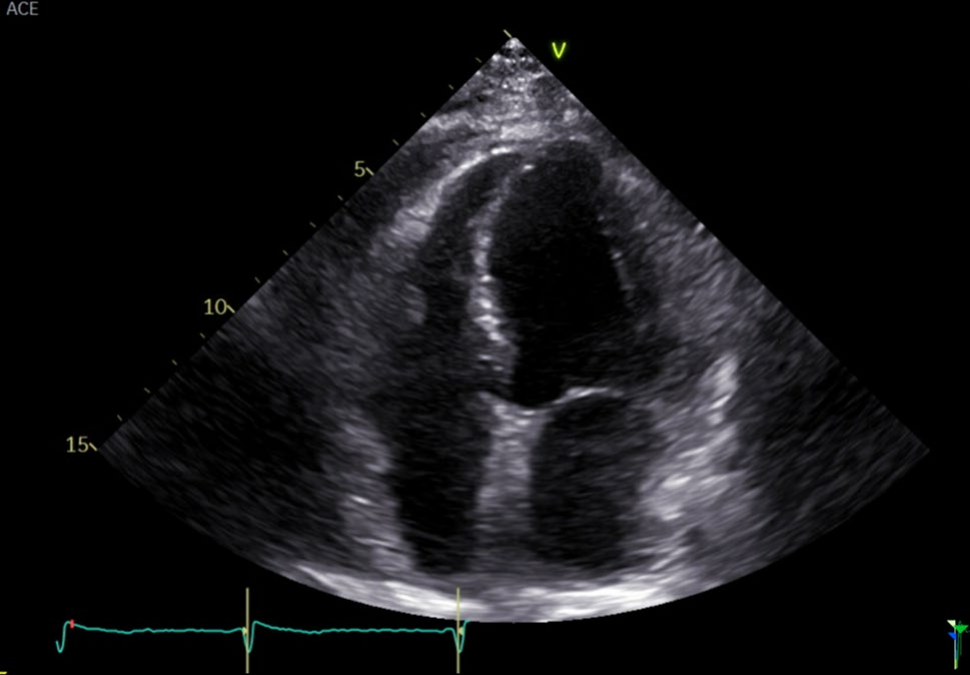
Echocardiography with mild concentric myocardial hypertrophy
